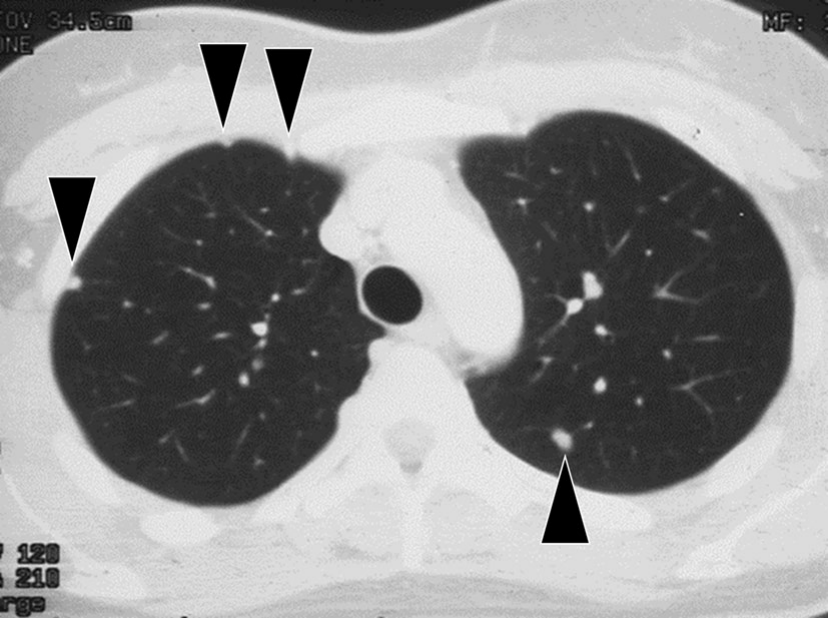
Preoperative image of the first relapse. Computed tomography revealing multiple pulmonary lesions (arrows) (a)
